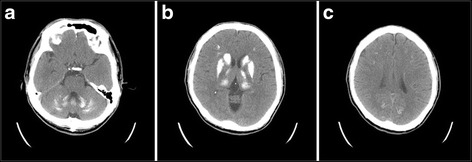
Axial noncontrast CT of the brain. Extensive and symmetric calcifications can be observed in a bilateral cerebellar dentate nuclei and hemispheres, b bilateral basal ganglia, internal capsules, and thalami, and c bilateral cerebral subcortical white matter
